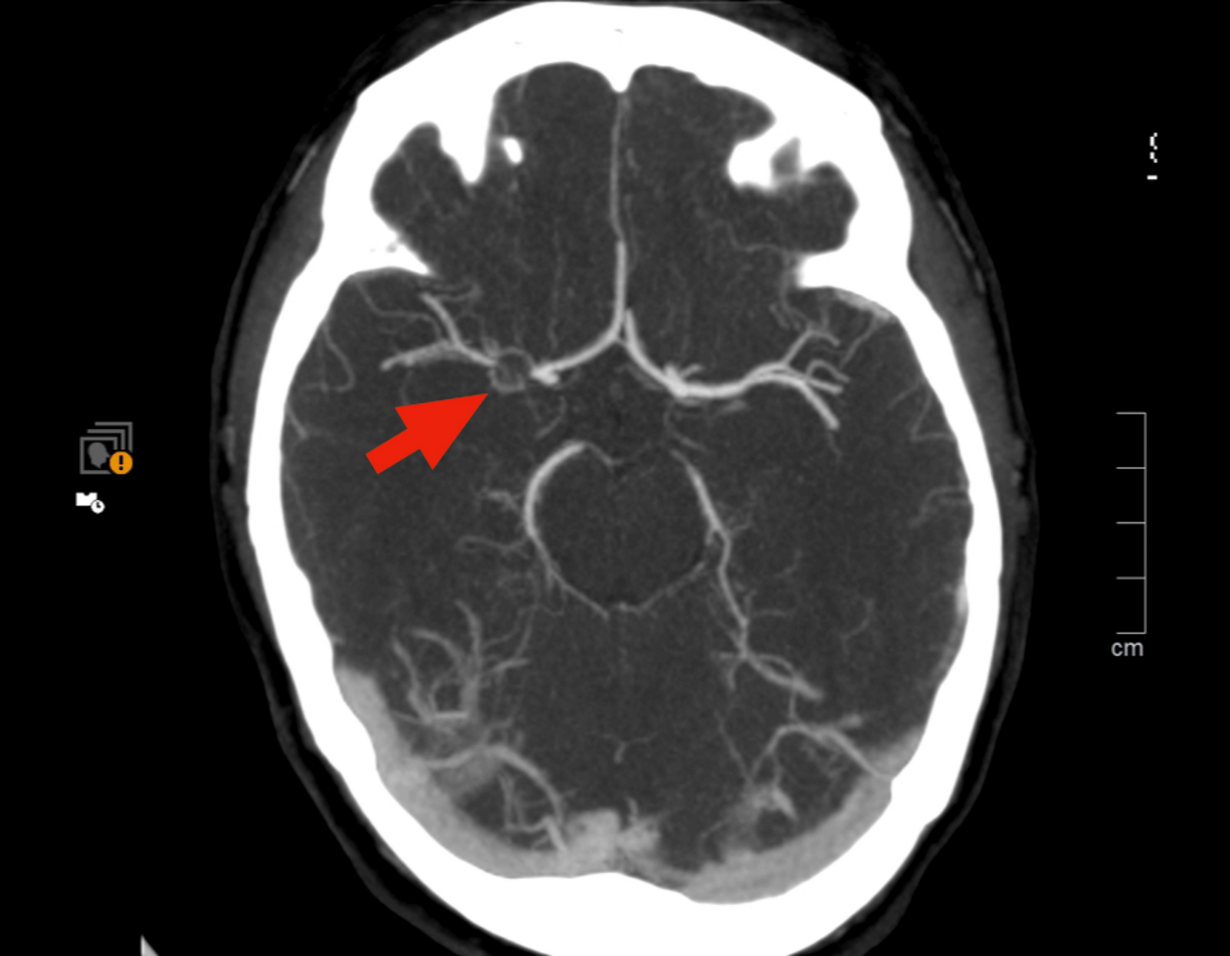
Cranial CT angiography shows a stop sign in the M1 segment of the right middle cerebral artery
